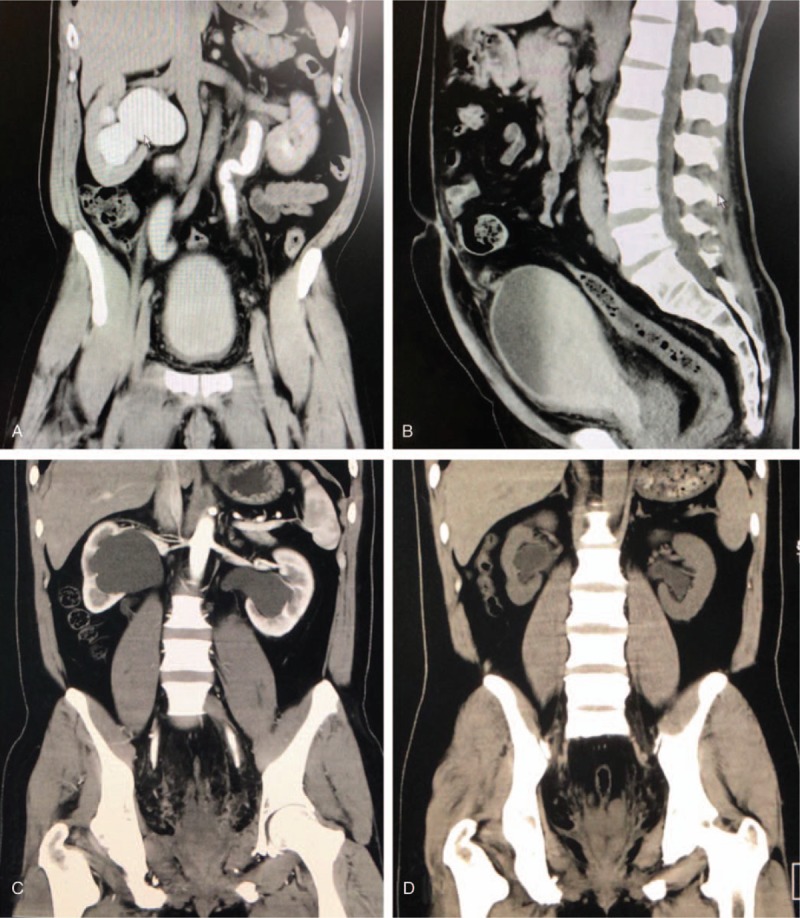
A, B, Pelvic computed tomography (CT) scans in coronal and sagittal planes showed that the bladder and sigmoid colon are compressed by an unencapsulated homogeneous soft tissue mass. C, D, Preoperative and postoperative CT scans of the same patient showed alleviation of bilateral hydronephrosis.
